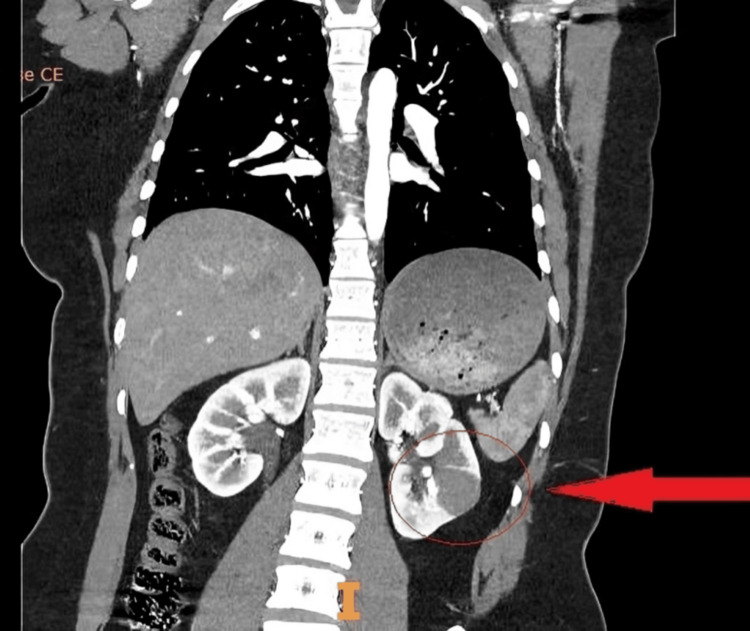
A Coronal view of CT thorax and abdomen with contrast showing a 2.8 cm left mid-pole renal lesion (red arrow and circle).
